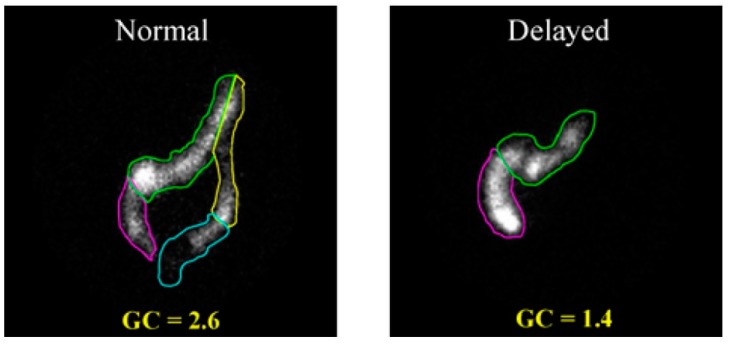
Colonic transit study images acquired with gamma scintigraphy. Reproduced with permission from [61]; published by Springer, 1979.
